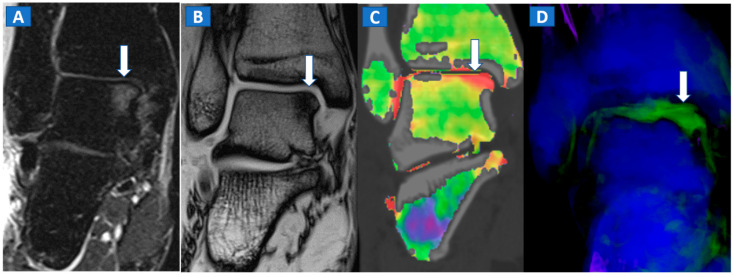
A 44-year-old male with post-traumatic OCL of the talar dome. On the coronal STIR MRI image (A), a hyperintense subchondral area of BME is depicted on the medial aspect of the talar dome (arrow), which is consistent with the diagnosis of OCL of the talus. The lesion appears hypointense on the T1-weighted images ((B); arrow). On the 1 mm reconstructed 2D coronal DECT image (C) and coronal 3D DECT map, the lesion appears as a subchondral oedematous area (arrow), due to its increased water content. On the corresponding 3D image (D) the BME is coded in green (arrow).
